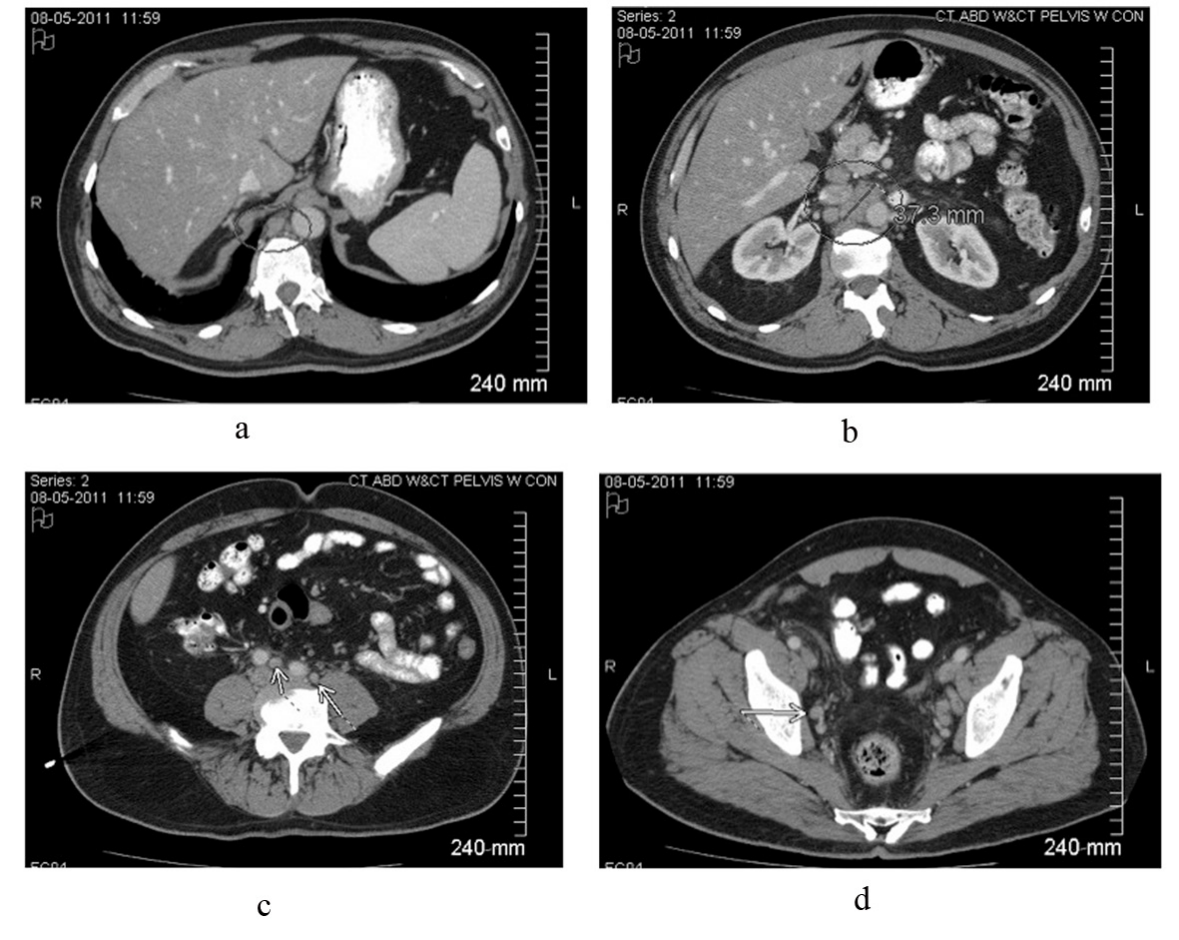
Contrast-enhanced CT scan of the abdomen and pelvis reveals intrabdominal and retroperitoneal adenopathy of uncertain origin. Adenopathy includes right retrocrural, retroperitoneal, celiac, peripancreatic, mesenteric, iliac and pelvic lymph nodes. Upper retroperitoneum reveals confluent nodal mass measuring 37.3 millimeters in image b.
